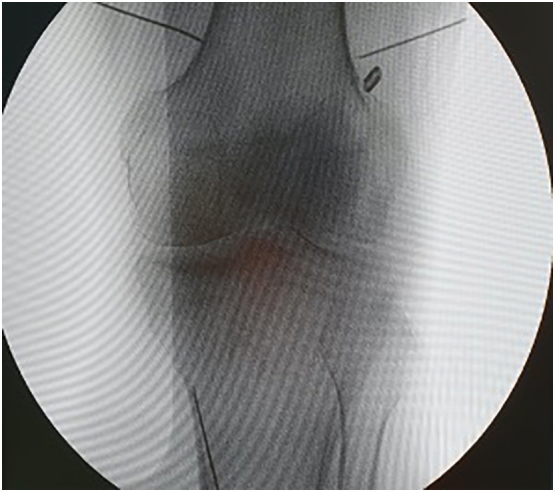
Fluoroscopic anteroposterior image after ultrasonography-guided needle insertion for genicular nerve block confirming placement of the cannula directed at the base of the medial and lateral femoral condyles and the base of the medial tibial.
